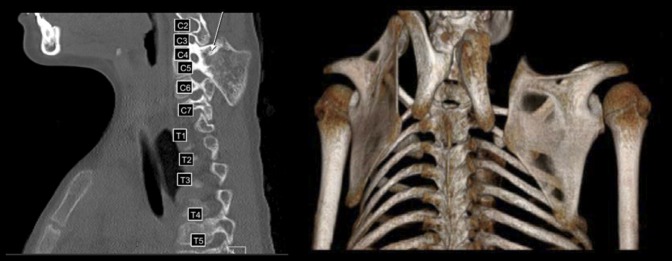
Photographs of two-dimensional CT sagittal view and three-dimensional CT view showing bilateral Sprengel deformities. Bilateral omovertebral bones, which proximally had an osseous connection to the scapula on the left side and fibrous on the right. Omevertebral bones are fused with posterior elements of the cervical spine.
